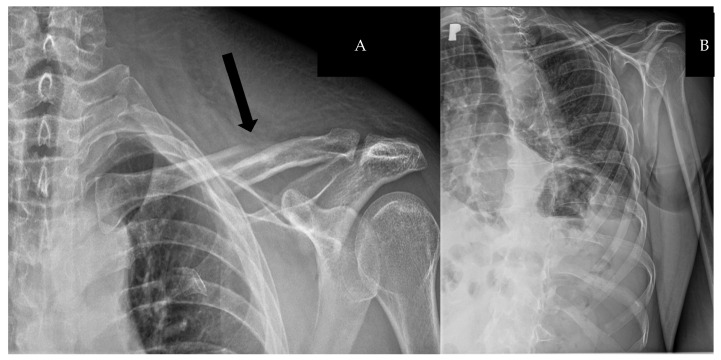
(A) Chest X-ray: oblique fracture of the clavicle shaft (black arrow). (B) Chest X-ray: flail chest with dual fractures of the second through seventh ribs on the left side.
